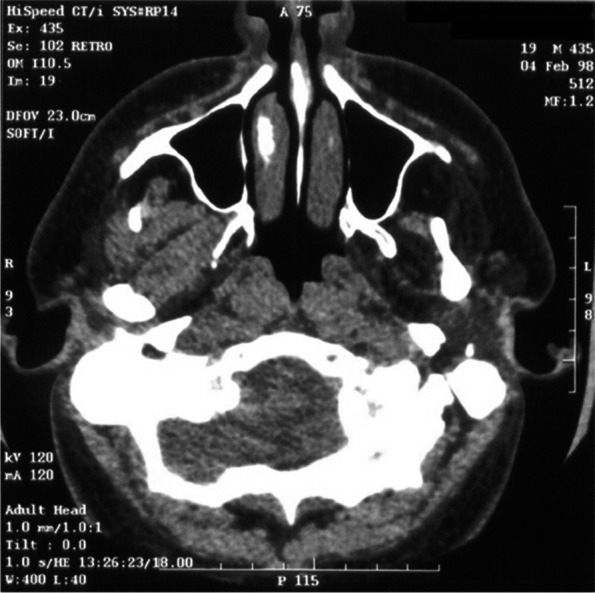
CT scans. The severe atrophy with fat substitution of the left internal and external pterygoid, left masseter and left anterior temporalis muscles is evident
